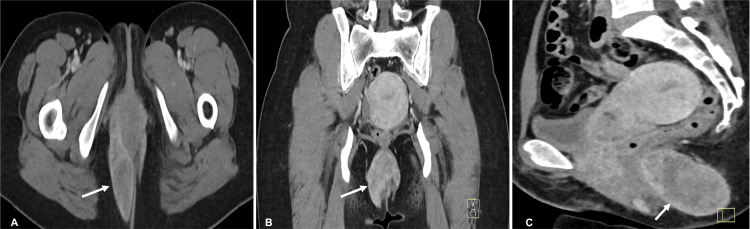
Contrast-enhanced CT scan of the pelvis. Axial (A), Coronal (B) and Sagittal (C) sections show a perianal solid mass lesion (blue arrow) extending across the anal sphincter showing heterogenous enhancement.
